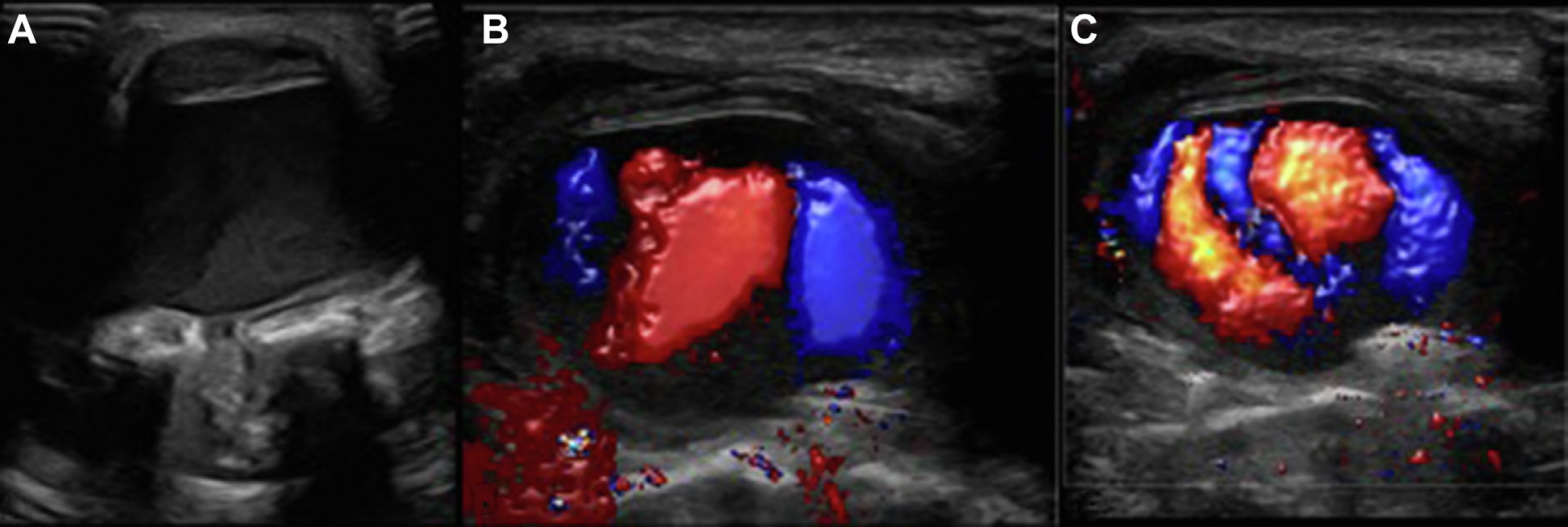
Ultrasound Images Ultrasound images on initial presentation of the upper sternal lump 2 months postoperatively. (A) B-mode imaging shows a wave at the inferior aspect of the sac indicating flow within the sac, (B and C) Color Doppler imaging demonstrates the yin/yang sign of swirling flow in a pseudoaneurysm.
